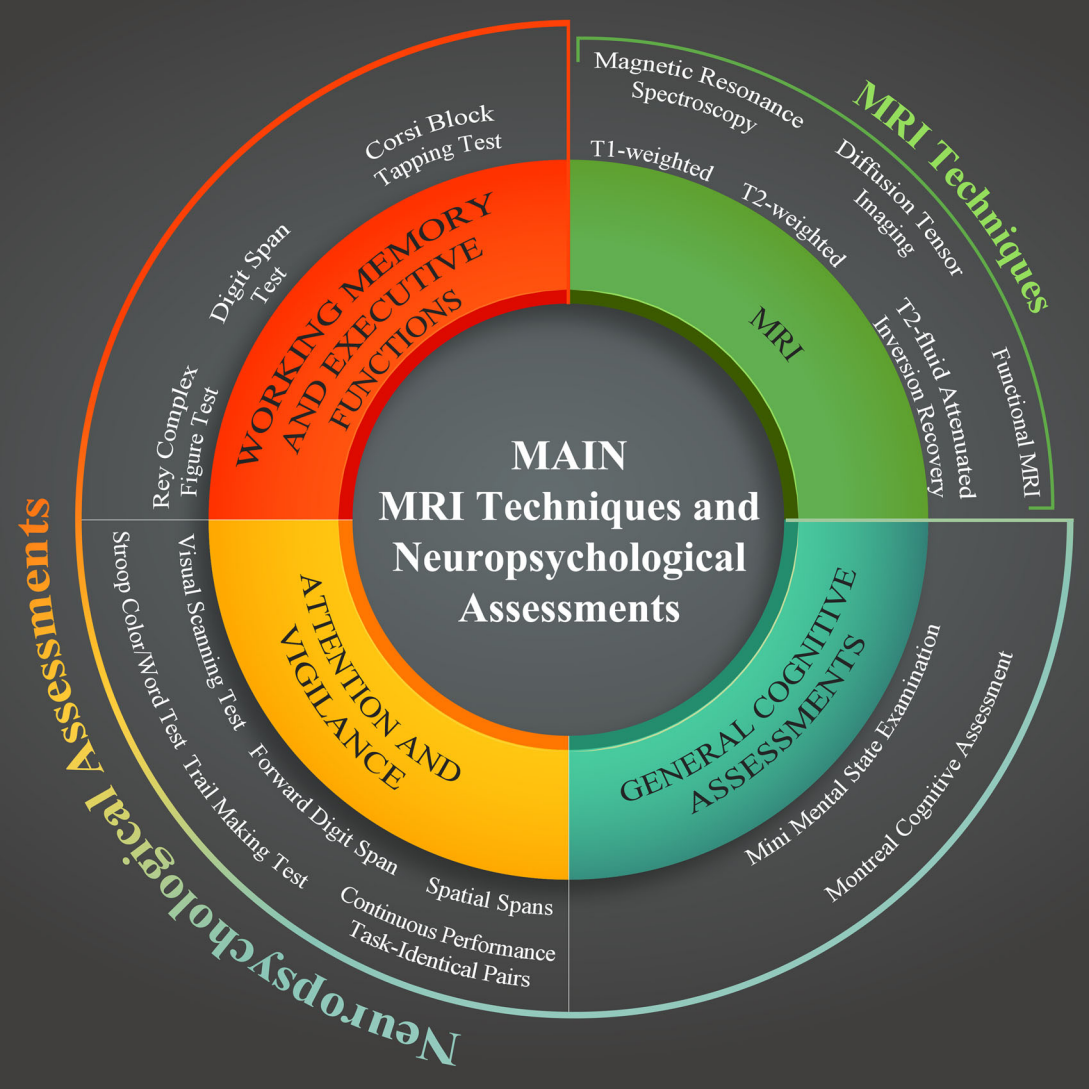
Main magnetic resonance imaging (MRI) techniques and neuropsychological assessments using the included studies.
